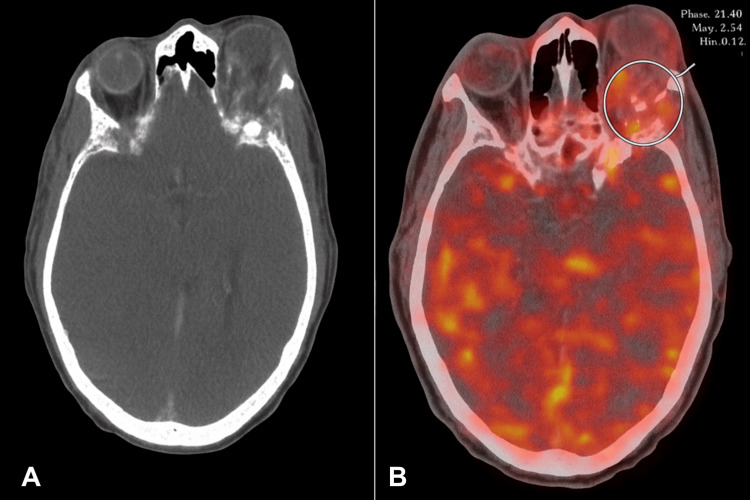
Axial CT (A) and fused PET/CT (B) correlating a destructive left spheno-orbital lesion with FDG avidity. The non-contrast axial computed tomography (CT) on the left reveals a destructive, expansile lesion involving the left spheno-orbital region. The fused positron emission tomography/computed tomography (PET/CT) on the right highlights marked fluorodeoxyglucose (FDG) uptake within the lesion (circled), confirming its hypermetabolic nature and supporting intracranial extension.
